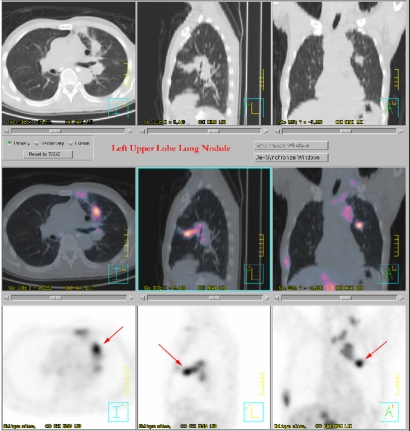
A PET-CT image can provide more accurate diagnostic and staging information on a lung tumour for IMRT treatment planning (courtesy of Dr. Hector Ma, St. Teresa's Hospital, Hong Kong)
